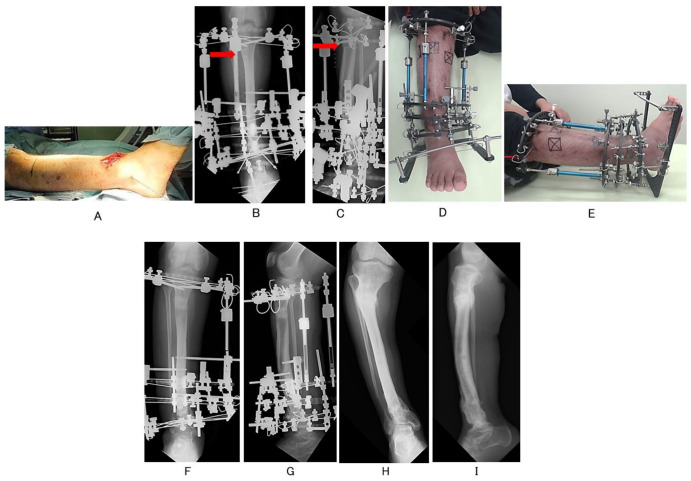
Surgical procedure and postoperative course at our institution. (A) Intraoperative photograph showing exposure and refreshment of the distal tibial nonunion site after removal of retained fixation material. (B,C) Immediate postoperative anteroposterior and lateral radiographs. Red arrows indicate the proximal osteotomy site for distraction osteogenesis. (D,E) Clinical photographs during the distraction phase. (F,G) Anteroposterior and lateral radiographs at 9 months postoperatively, showing regenerate formation after completion of lengthening. (H,I) Anteroposterior and lateral radiographs at the latest follow-up, demonstrating bony continuity of the docking site and distraction regenerate, mild residual varus deformity of the proximal tibia, and durable limb preservation.
